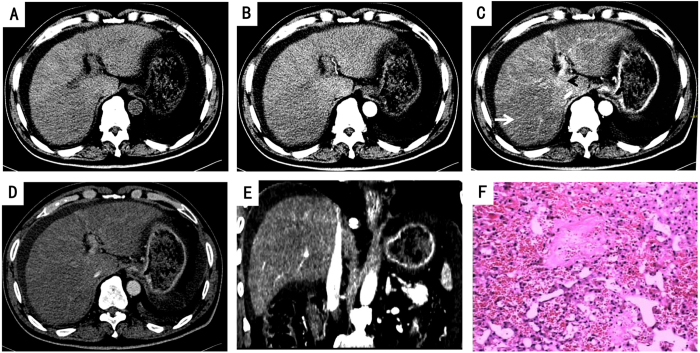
67-year-old man diagnosed with gynura segetum-induced HSOS received contrast-enhanced CT scan and liver biopsy. (A–D) Image of plain and contrast-enhanced CT scan; (A) plain CT scan; (B) arterial phase; (C) portal phase; (D) equilibrium phases. The CT image in portal phase showed patchy liver enhancement (arrowhead,) and heterogeneous hypoattenuation (arrow) in PAs-induced HSOS. (E) Multiple planar reconstruction CT angiogram shows a normal patent inferior vena cava despite it was slightly compressed by swelling liver. (F) Pathologic examination showed that demonstrates sinusoidal congestion and dilation, and extensive extravasation of erythrocytes into pericentral parenchyma.
